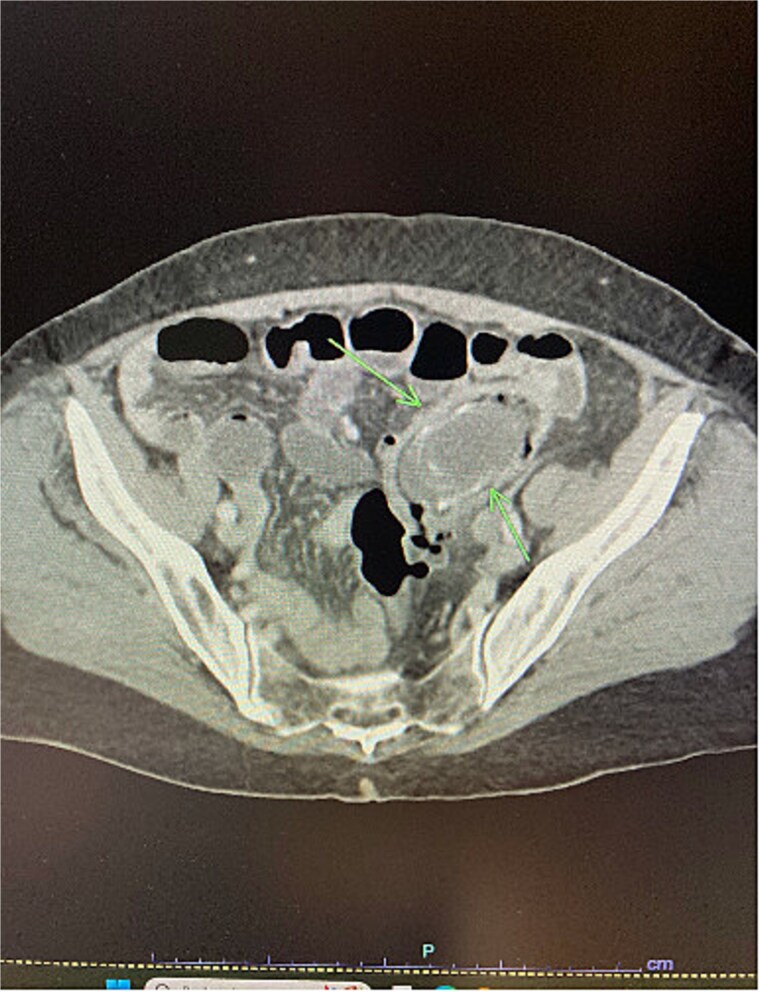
Arrows showing gallstone impacted in the proximal sigmoid colon on CT scan.
